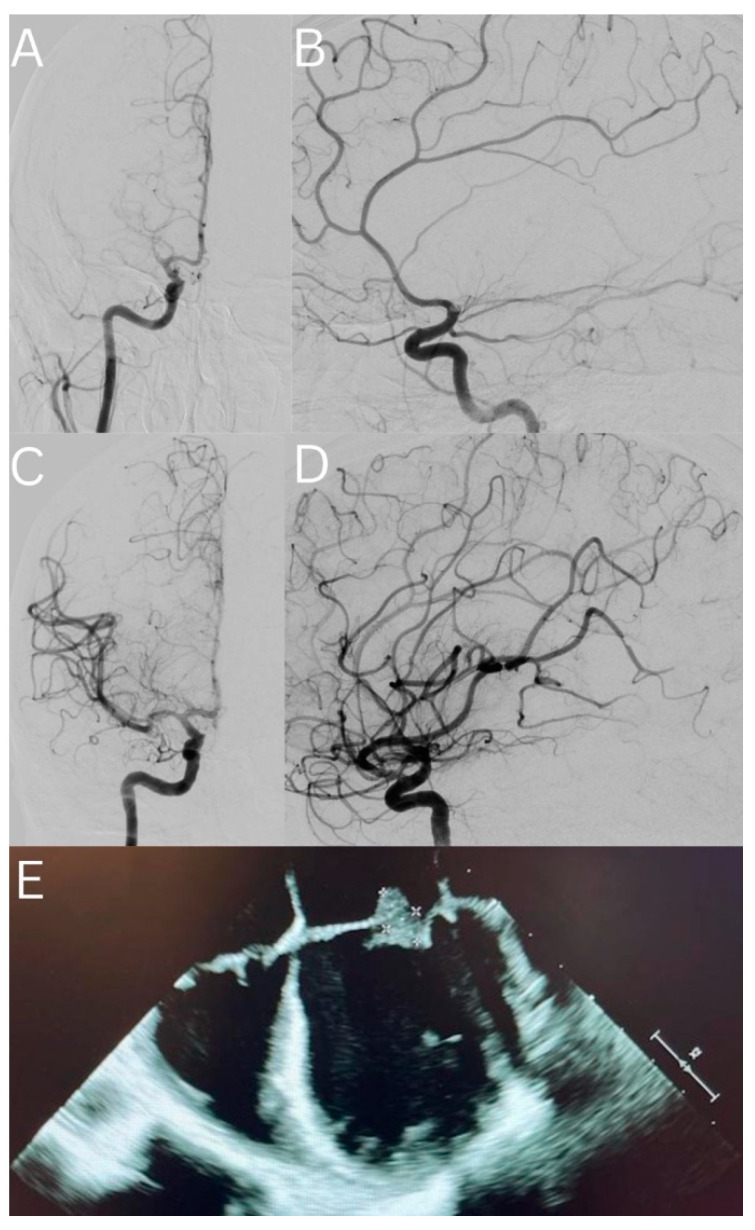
(A) Pre-op right internal carotid artery (ICA) angiogram (AP view), which shows right MCA (proximal M1 segment) occlusion; (B) pre-op right ICA angiogram (lateral view), which shows right MCA (proximal M1 segment) occlusion; (C) post-op right ICA angiogram (AP view), which shows complete re-canalization of the right MCA (TICI score of 3); (D) post-op right ICA angiogram (lateral view), which shows complete re-canalization of the right MCA (TICI score of 3); (E) transesophageal echocardiogram of the patient demonstrating a mobile vegetation on the mitral valve.
